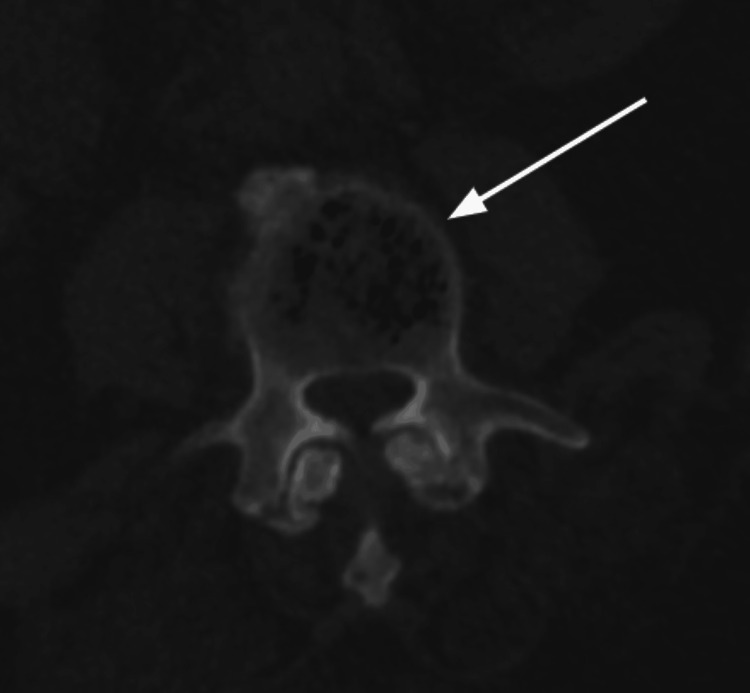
CT image-guided biopsy visualization of the “pumice stone sign” in the L3 vertebra corresponding with emphysematous osteomyelitis.
